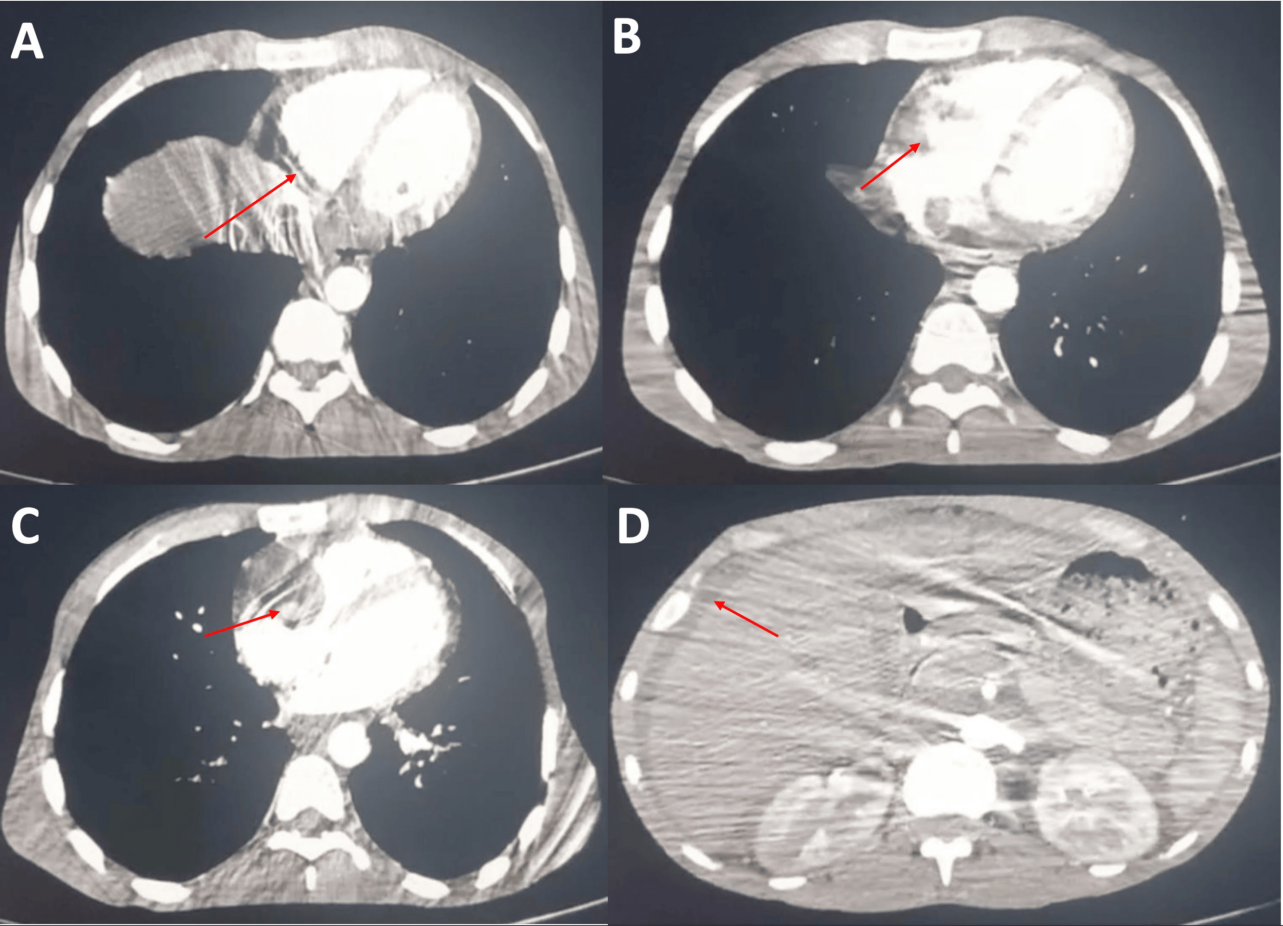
Abdominal CT: Extensive IVC thrombus with hepatic congestion and massive ascites. (A) Extensive thrombus within the supra-hepatic IVC (arrow). (B) Thrombus extending cranially toward the right atrium (arrow). (C) Irregular filling defect at the right atrium contiguous with the IVC thrombus(arrow). (D) Hepatic congestion with large-volume ascites. (arrow). Images were obtained from the authors’ institutional database. IVC, inferior vena cava
